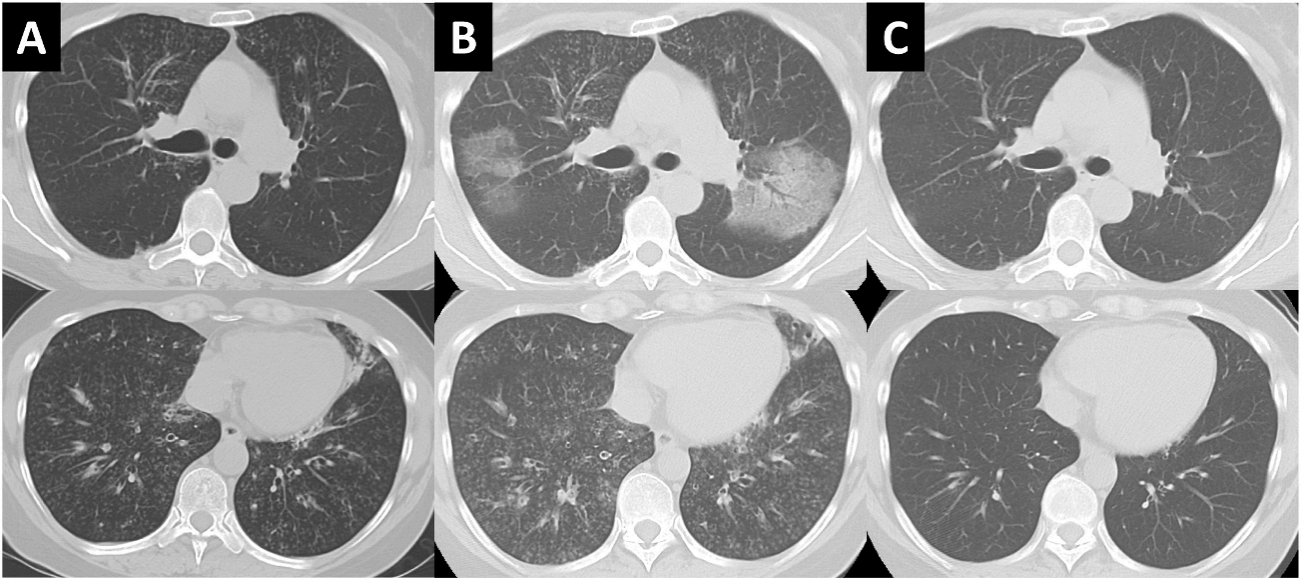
(A) Chest computed tomography (CT) scan at first examination showing diffuse centrilobular nodules and bronchial wall thickening. (B) Pre-treatment chest CT scan after bronchoscopy showing a diffuse nodular shadow and new ground-grass opacity in the right middle and left lower lobes. (C) Two months after the initiation of treatment, chest CT scan showed that both the ground-grass opacity and nodular shadow had disappeared.
